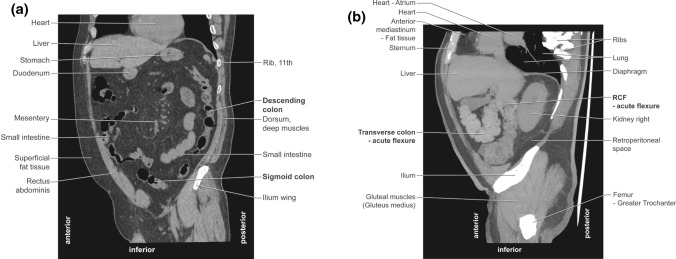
CT scans (tracing—main features). Subject with 4 acute flexures of the colon. Patient BW. F, age 69 years, weight 60 kg, height 164 cm, BMI 22.3. Date of examination: 18.08.2018. A Sagittal plane. Very straight course of the colon. No acute flexures on the scan. B Sagittal plane. Two acute colon flexures are shown on the scan (1 in the RCF, 1 in the transverse colon)
